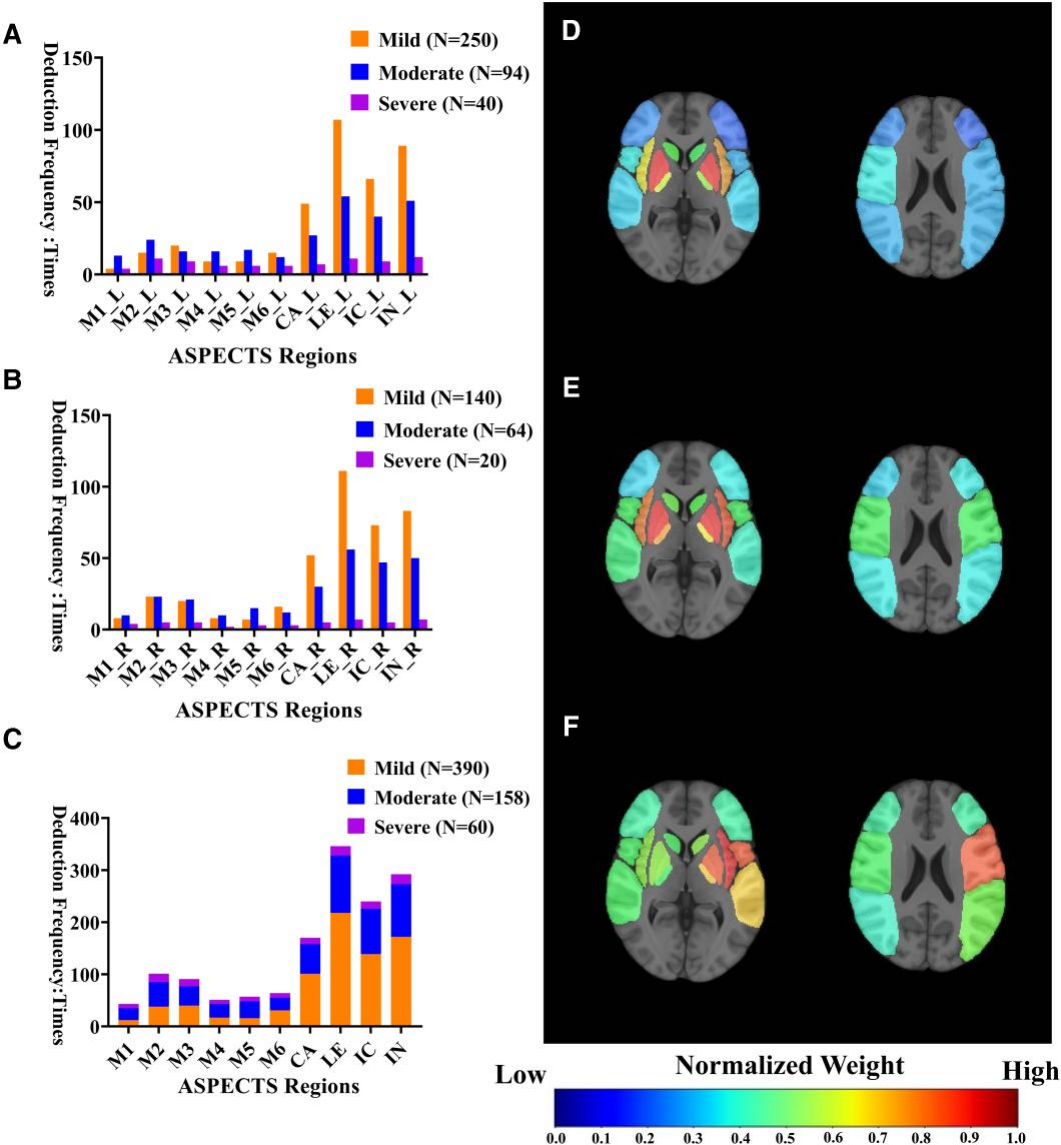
The cumulative histogram of deduction frequency in ASPECTS regions for MCA-AIS with different degrees: (A) patients with left MCA-AIS, (B) patients with right MCA-AIS and (C) all the included patients. The normalized deduction frequency chart of ASPECTS regions in MCA-AIS with different degrees, the colour bar scale from 0 to 1 represents the deduction frequency from low to high: (D) Mild, (E) moderate and (F) severe. *N* = Numbers of patients, ASPECTS = the Alberta Stroke Programme Early CT Score, CA = Caudate, LE = Lentiform_nucleus, IC = Internal_capsule and IN = Insular_ribbon.
